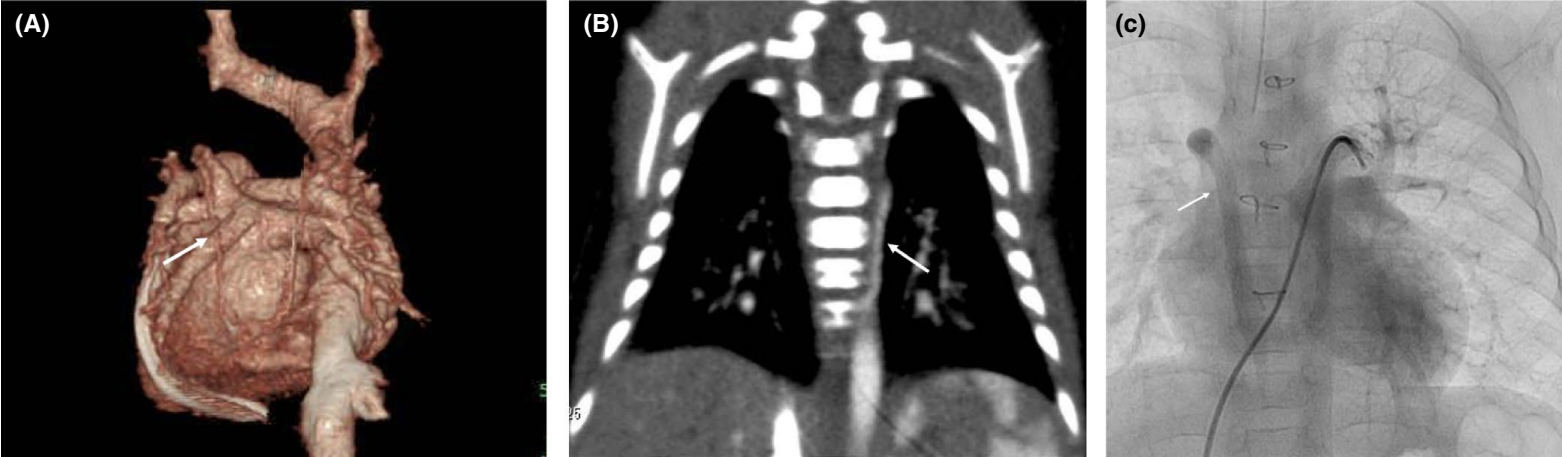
A, Contrast CT after surgery repair (behind view). White arrow indicates second vertical vein (originated from proximal ligated vertical vein and drain into superior vena cava). B, White arrow indicates accessory hemiazygos vein. C, Left pulmonary angiogram. White arrow indicates second vertical vein into superior vena cava
